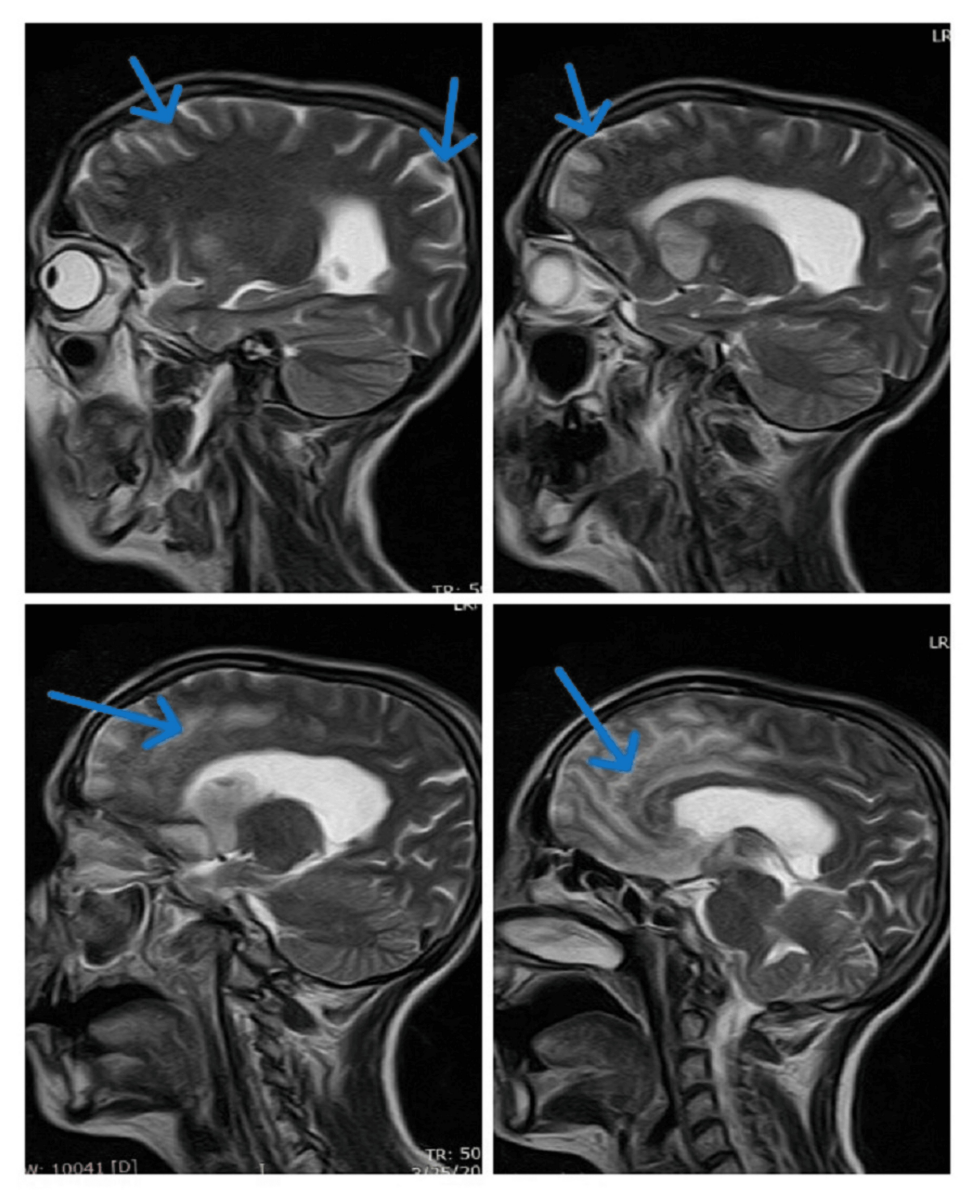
T2-weighted imaging (T2WI) MRI (sagittal view) shows multifocal ischemia (blue arrows)
